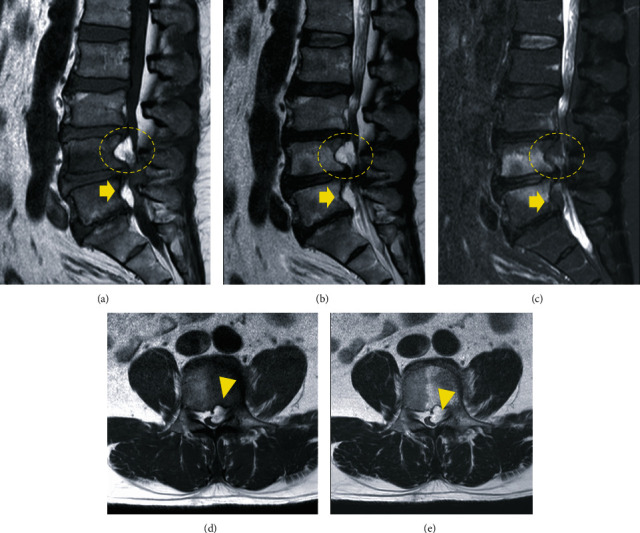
Magnetic resonance imaging on T1-weighted (a), T2-weighted (b), and T2-fat suppression (c) reveal a mass (circles) of high-signal intensity at the L4 pedicle level in the left epidural space. The following were observed: a hyperintense anterior epidural mass (arrows) with strangulation of the dural sac at L4-5 levels (the first row), epidural mass (arrowheads) at L4 compressed asymmetrically on the left side of the cauda equina, and exiting left L4 nerve root on axial T1- and T2-weighted images (d, e) (the second row).
